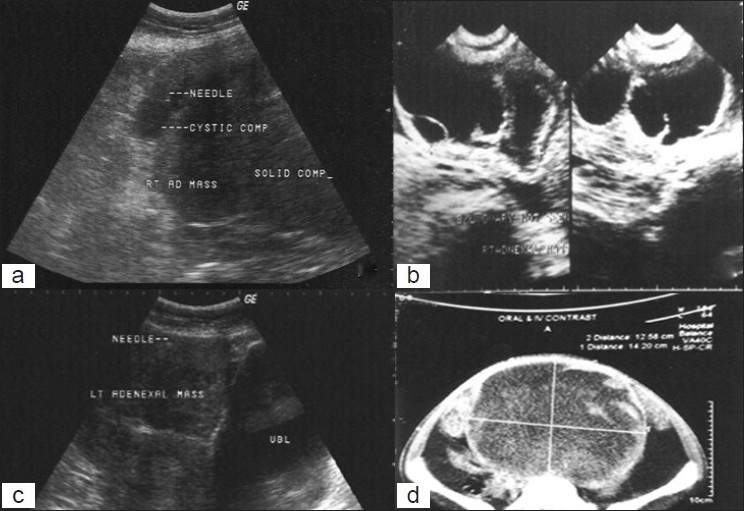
Ultrasonography scan showing (a) right adnexal mass (serous cystadenoma) with solid and cystic components (b) solid cystic mass involving both ovaries (bilateral serous cystadenocarcinoma) and (c) left adnexal mass with needle inside the lesion (d) contrast-enhanced computed tomography: large ovarian mass of mixed fat and soft tissue attenuation (teratoma)
